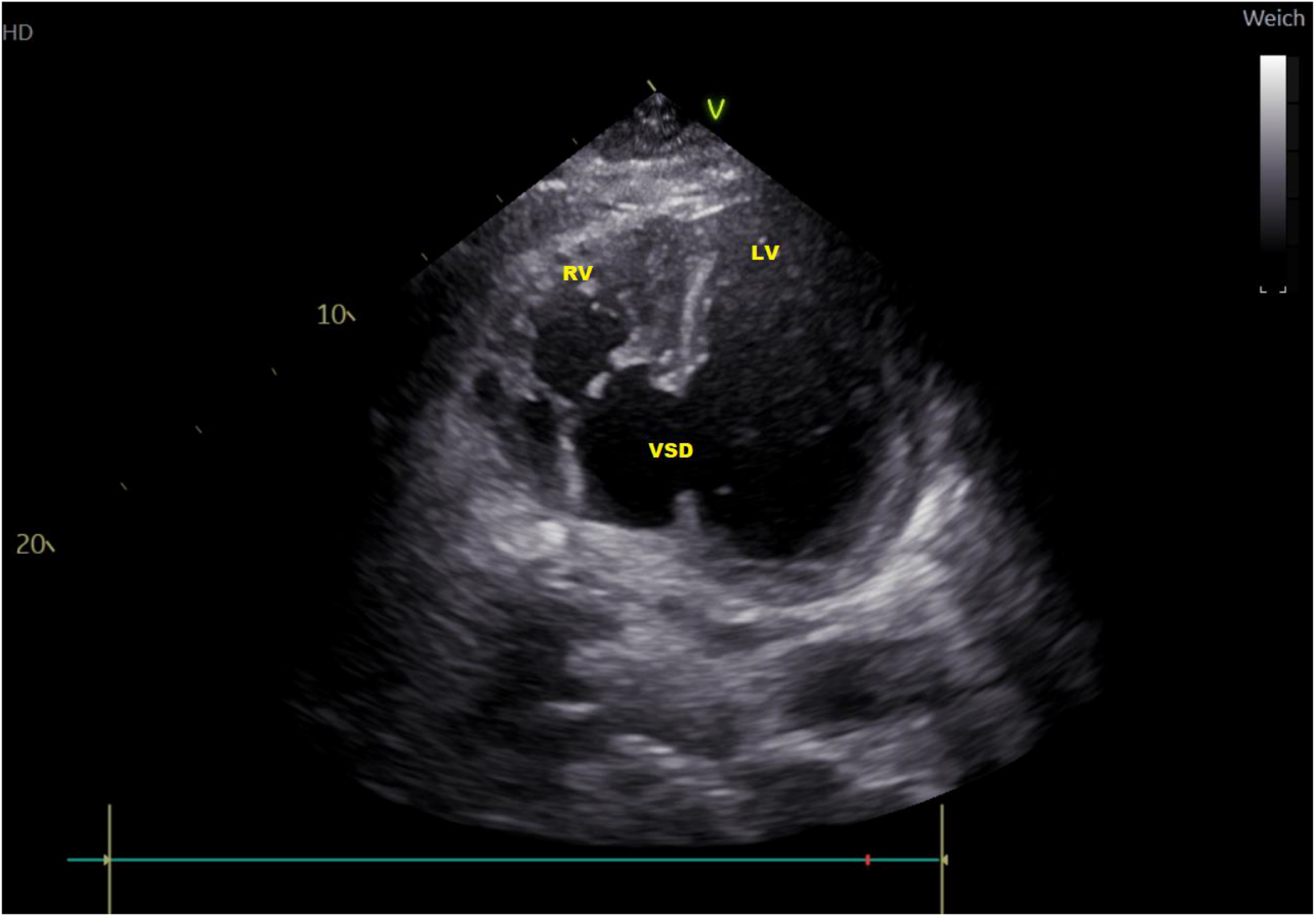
Transthoracic echocardiography atypical two-chamber view: ventricular septal defect (VSD), loaded left and right ventricle (LV, RV).
